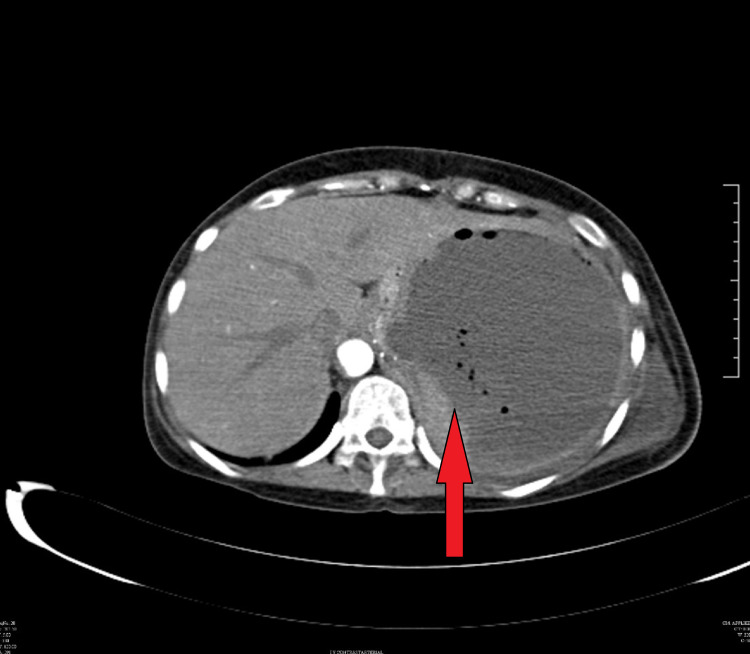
CT abdomen showing splenic abscess with air foci.
